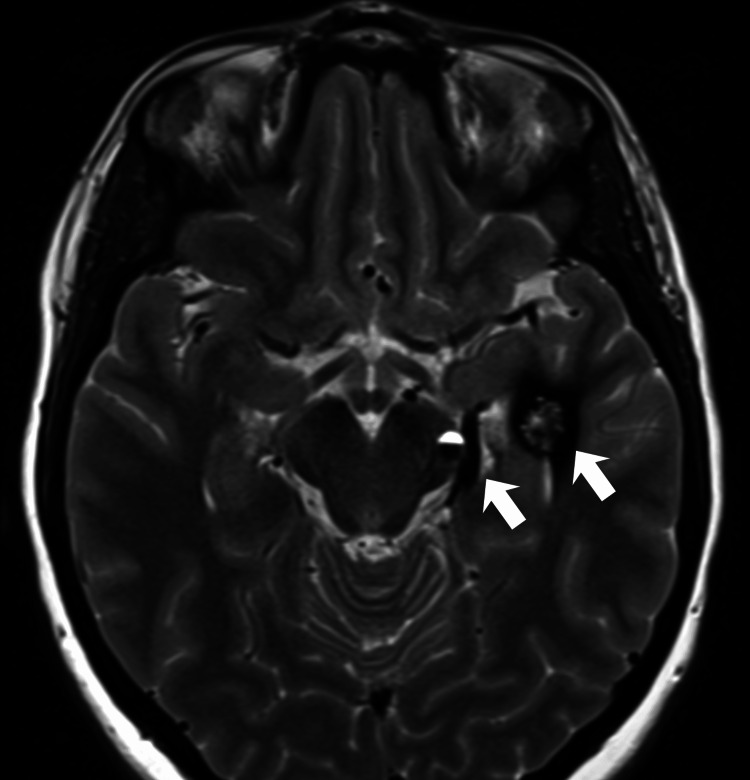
Temporal lobe cavernoma and associated DVA T2-weighted image showing a typical cavernous malformation in the temporal lobe, a dilated basal vein in the lateral basal cistern, and a mixed-signal brainstem lesion with hemorrhage. DVA: developmental venous anomalies
